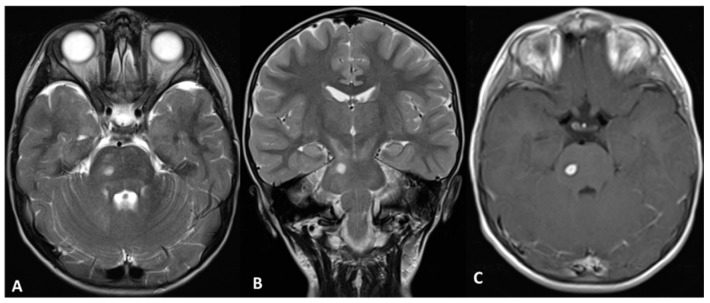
Brainstem Glioma (Subject 05 CT). (A,B) Axial and Coronal T2 weighted images (w.i.); (C) axial T1 w.i. after gadolinium administration show diffuse abnormal signal intensity in the pons and a spot of circumscribed hyperintensity with enhancement consistent with a low-grade glioma. CT: Central nervous system tumor.
